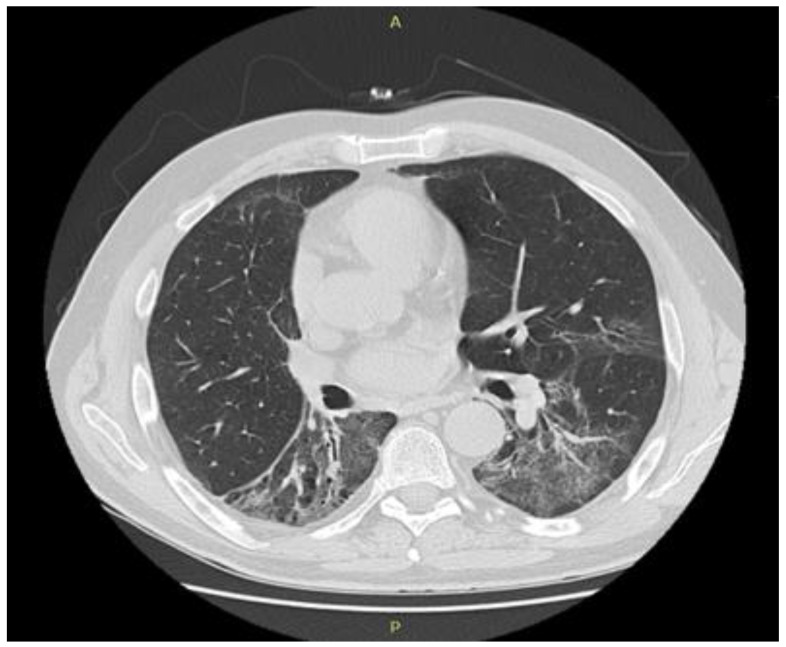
Chest computed tomography scan after 1 month of corticosteroid therapy: partial resolution of the interstitial infiltrates.
